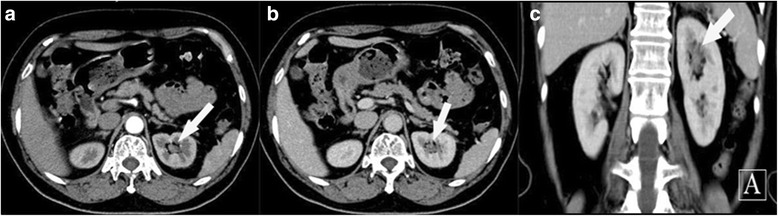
Enhanced abdominal computed tomography revealing a soft tissue density (*white arrow*) in the upper calyx of the left kidney (a) Cross section, arterial phase (b) Cross section, venous phase (c) Coronal plane
